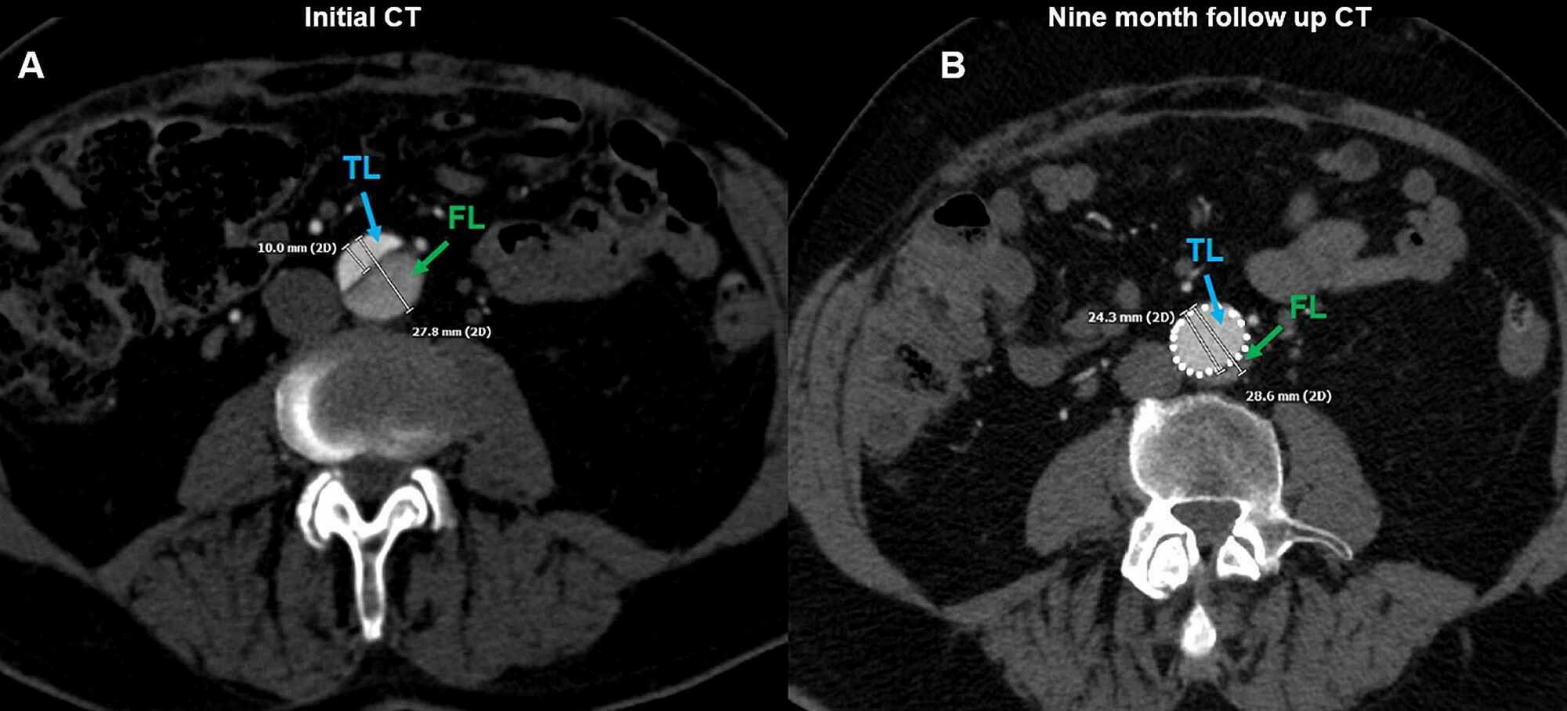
Mid-Term Surveillance True Luminal Patency of Thoracic Endovascular Aortic Repair The initial pre-procedural axial CT angiogram (Figure 3) shows the caliber sizes of the respective true (blue arrow) and false (green arrow) dissection lumens (A). The aortic diameter measures 27.8 millimeters in diameter at this level. As demonstrated (Figure 3) the false lumen measures 17.8 millimeters and is noticeably larger than the true lumen, measuring 10 millimeters. A comparison nine-month post-procedural axial CT angiogram of the same patient shows the caliber sizes of the respective true (blue arrow) and false (green arrow) dissection lumens after thoracic endovascular aortic repair with the covered and uncovered stent hybrid technique (B). The true lumen is noticeably larger in diameter than the false lumen, measuring 24.3 millimeters. The open cell/bare metal stent continues to exclude the false lumen after nine months.
